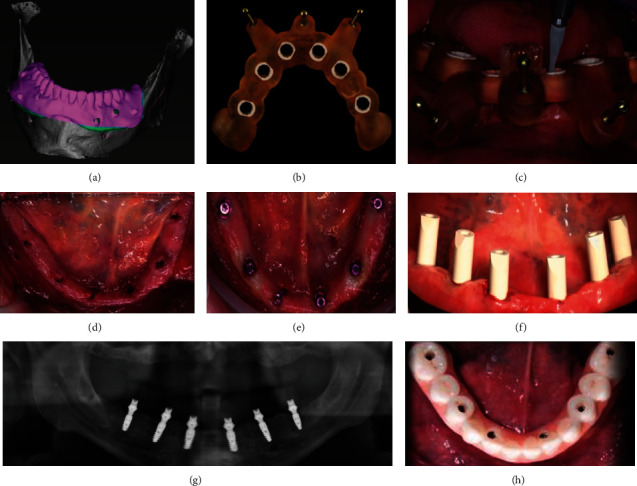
An implant-supported 3D-printed fixed denture was designed via CAD software (a). Meanwhile, a surgical stent was also printed (b) and used to guide the insertion of implants (c–f); the panoramic X-ray showed that the implants were well positioned (g); the printed fixed denture was subsequently delivered to the patient (h). Reprinted from [157].
